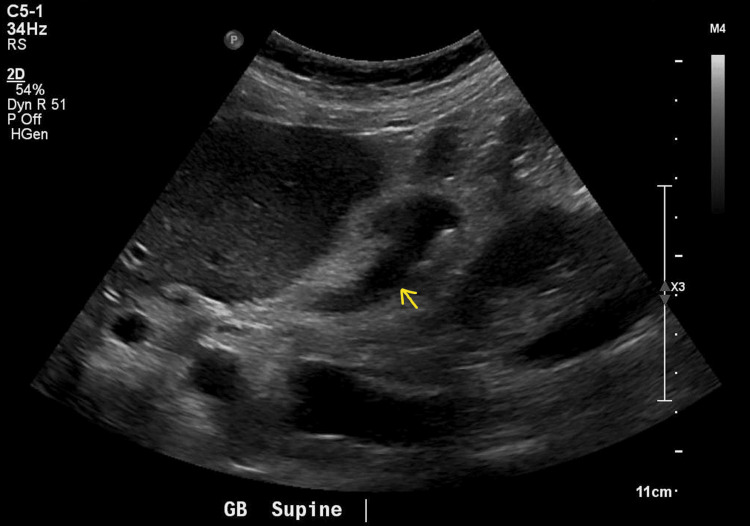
Abdominal ultrasound: contracted gallbladder with suspected cholelithiasis. No additional findings to suggest cholecystitis or biliary obstruction
